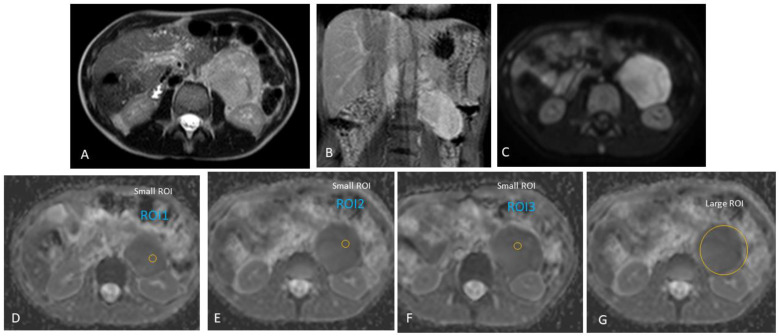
Left retroperitoneal ganglioneuroblastoma in an 11-year-old boy, presenting with an abdominal mass incidentally diagnosed on US. Homogenous solid tumour. Therefore, the regions-of-interest (ROIs) were randomly placed within the tumour during the analysis of apparent diffusion coefficient (ADC) diffusion-weighted (DW) MRI values. (A) Axial T2, (B) coronal post-gadolinium T1, and (C) axial DWI (b-value: 500 mm^2^/s). (D–F) Three small regions-of-interest (ROIs) on the ADC map with an average area of 15.3 mm^2^; ADC for the small ROI method, 1.05 × 10^−3^ mm^2^/s. (G) One large ROI on the ADC map with an average area of 1823.4 mm^2^; ADC for the large ROI method, 1.07 × 10^−3^ mm^2^/s.
